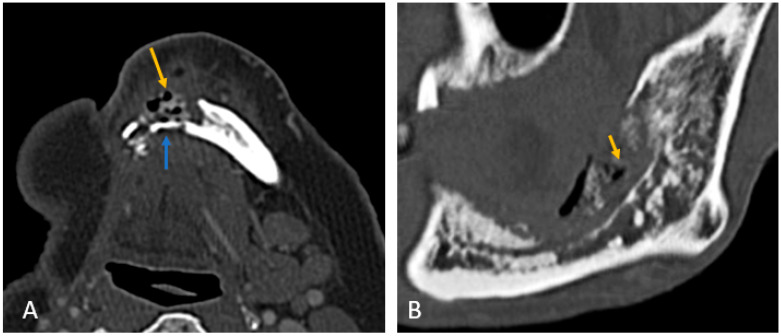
(A) Axial CT image of focal ORN along the resected margin in a case of segmental mandibulectomy. (B) Sagittal CT image of diffuse ORN in case of marginal mandibulectomy. There is bone resorption, coarse, sparse trabeculae, destruction of cortex, fragmentation (blue arrow), and intraosseous gas (orange arrow) present.
